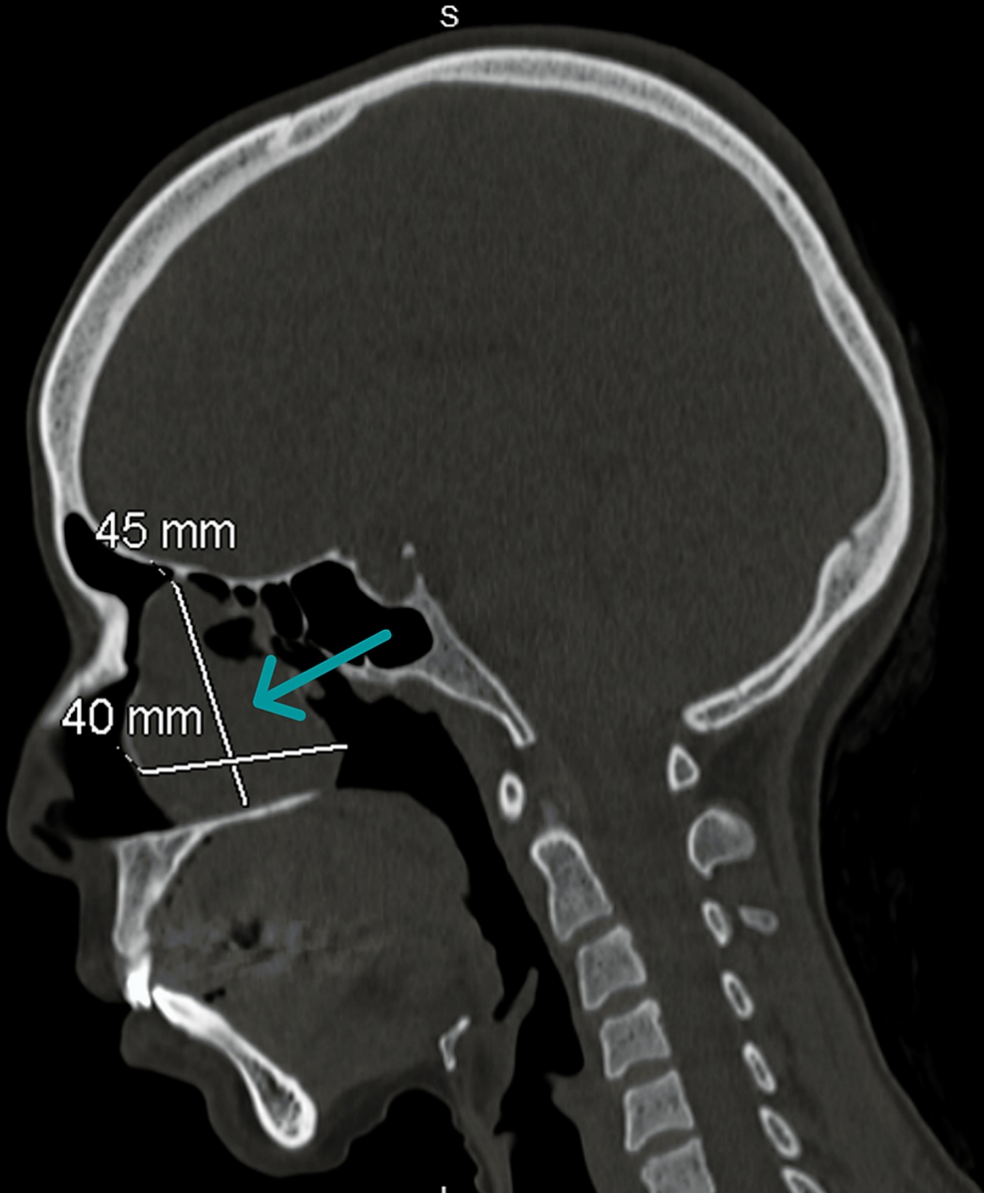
Preoperative CT of the paranasal sinus The sagittal view shows the measurement of a concha bullosa mucocele.
